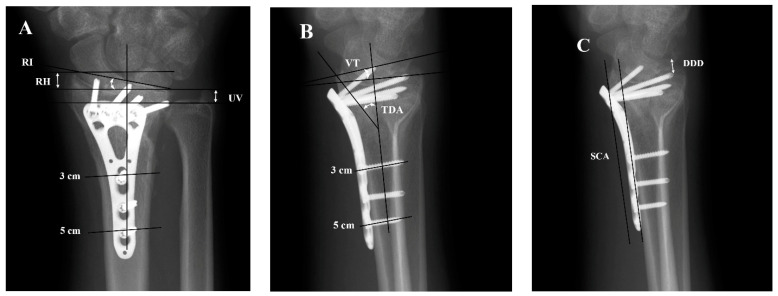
Radiographs of posteroanterior (A) and lateral view (B,C) of distal radius fracture with variable-angle volar locking plate. RH = Radial height, UV = Ulnar variance, RI = Radial inclination, VT = Volar tilt, TDA = Tear drop angle, DDD = Distal dorsal cortical distance, SCA = Soong classification auxiliary line.
